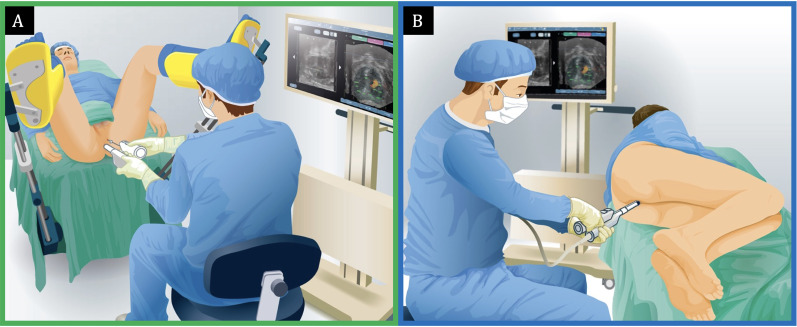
Prostate biopsy setup and templates. A) MRI/TRUS fusion-guided free-hand transperineal prostate biopsy. In this procedure, the biopsy gun is inserted through a coaxial needle, minimizing multiple punctures through the perineal skin. The patient remains in the supine position during the entire procedure. B) MRI/TRUS fusion-guided transrectal prostate biopsy. The prostate is sampled by inserting the needle through the rectum, with the patient placed in the left lateral decubitus position.
